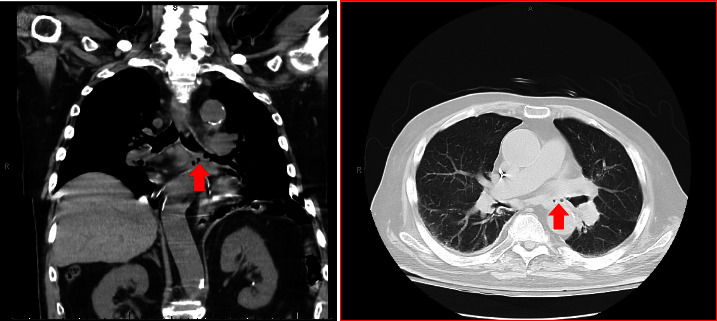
CT image without contrast of the patient's chest. Arrows indicating extraluminal air tracking from the left mainstem bronchus towards the esophagus.
